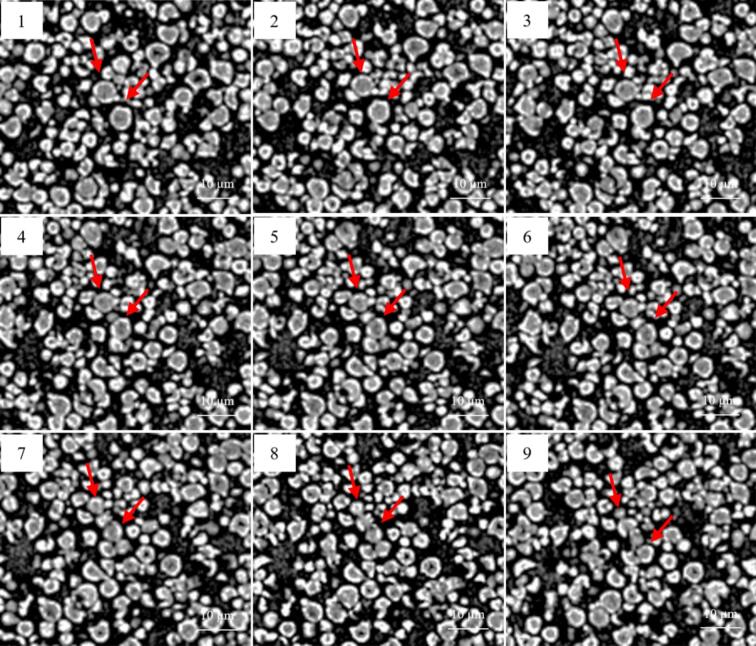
3D CT scanning of *Fagopyrum tataricum* starch-quercetin.
